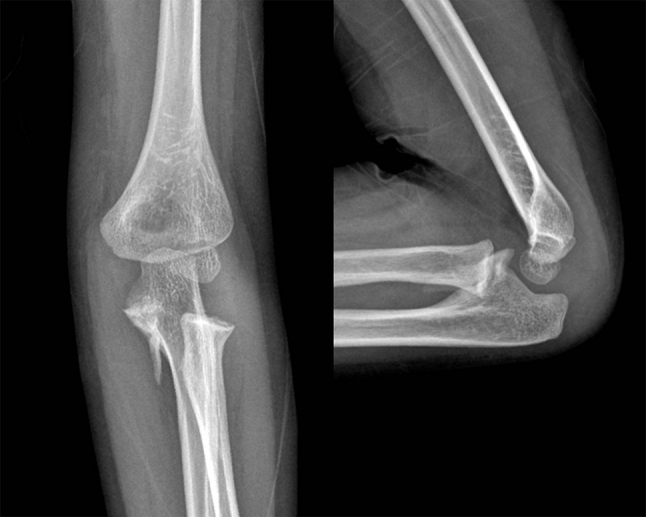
Radiographs at 6 months after the injury show well reduced elbow with calcification at proximal ulna
